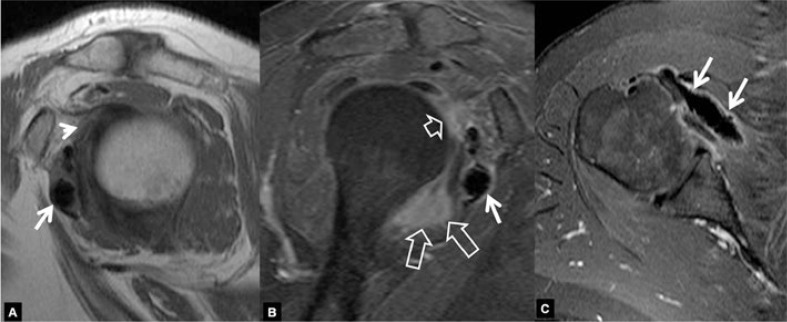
A 58-year-old female patient with a history of hydroxyapatite deposition disease over the subscapularis tendon and second ray adhesive capsulitis. The oblique sagittal T1-w (a) and fat suppressed contrast enhanced T1-w oblique sagittal (b) and axial (c) MR images show the calcific tendinopathy (arrows, a,b,c), the abnormal signal in the rotator cuff interval (arrowhead a) and the enhancement located in the rotator cuff interval (short open arrow) and in the axillary recess (long open arrows).
